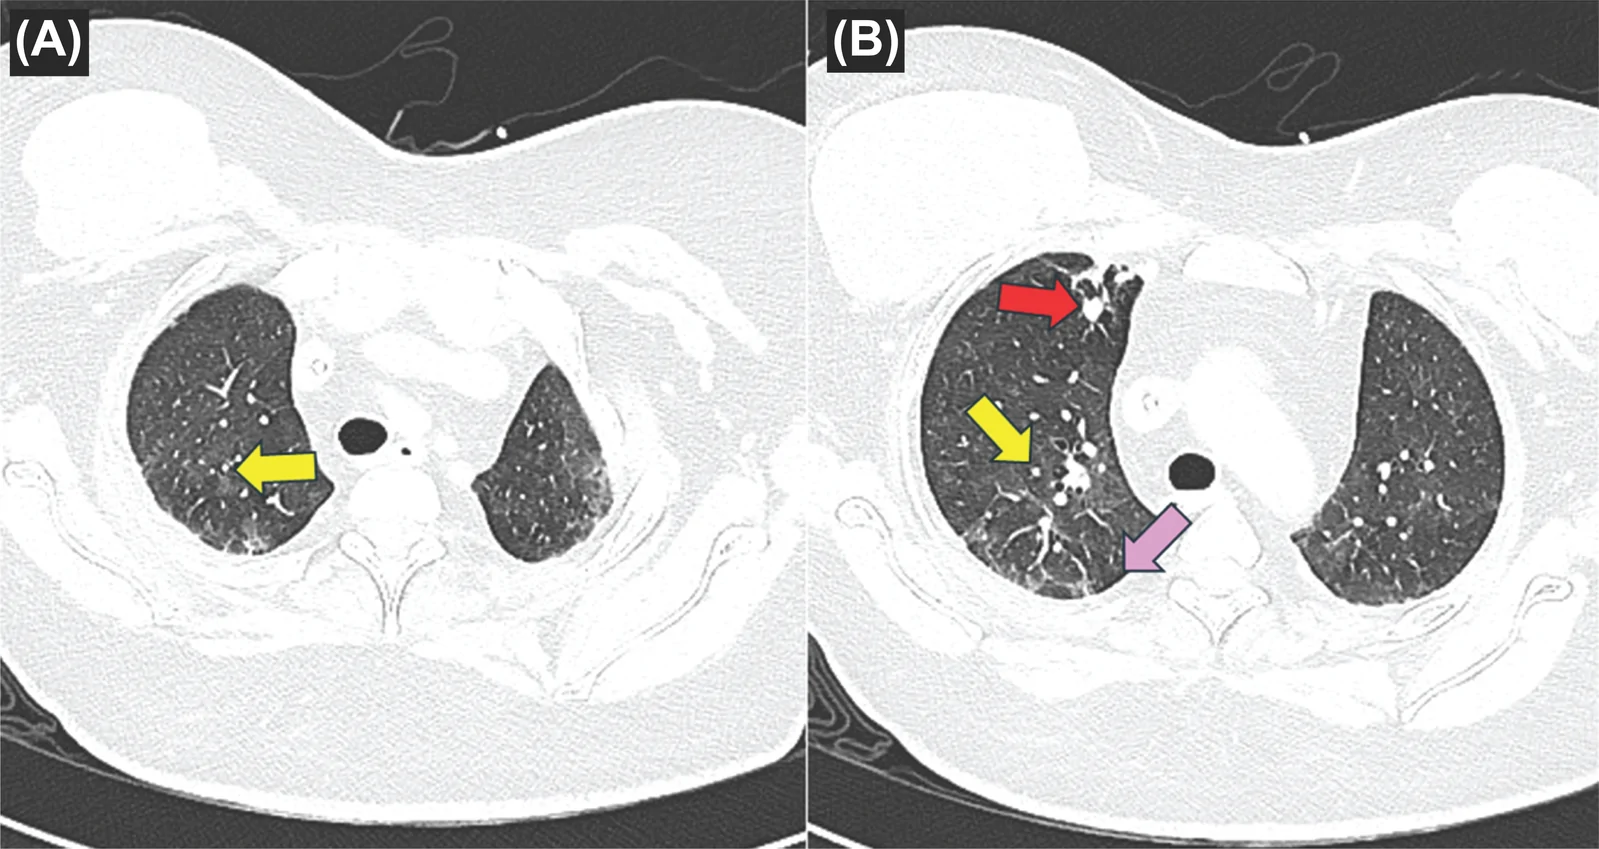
Chest CT, lung window (A, B). Ground-glass opacities in upper lobes and upper segments of lower lobes (yellow arrows); 1-cm cavitary nodule in the right upper-lobe apical segment (red arrow); subsegmental atelectasis (purple arrow). Abbreviation: CT, computed tomography.
